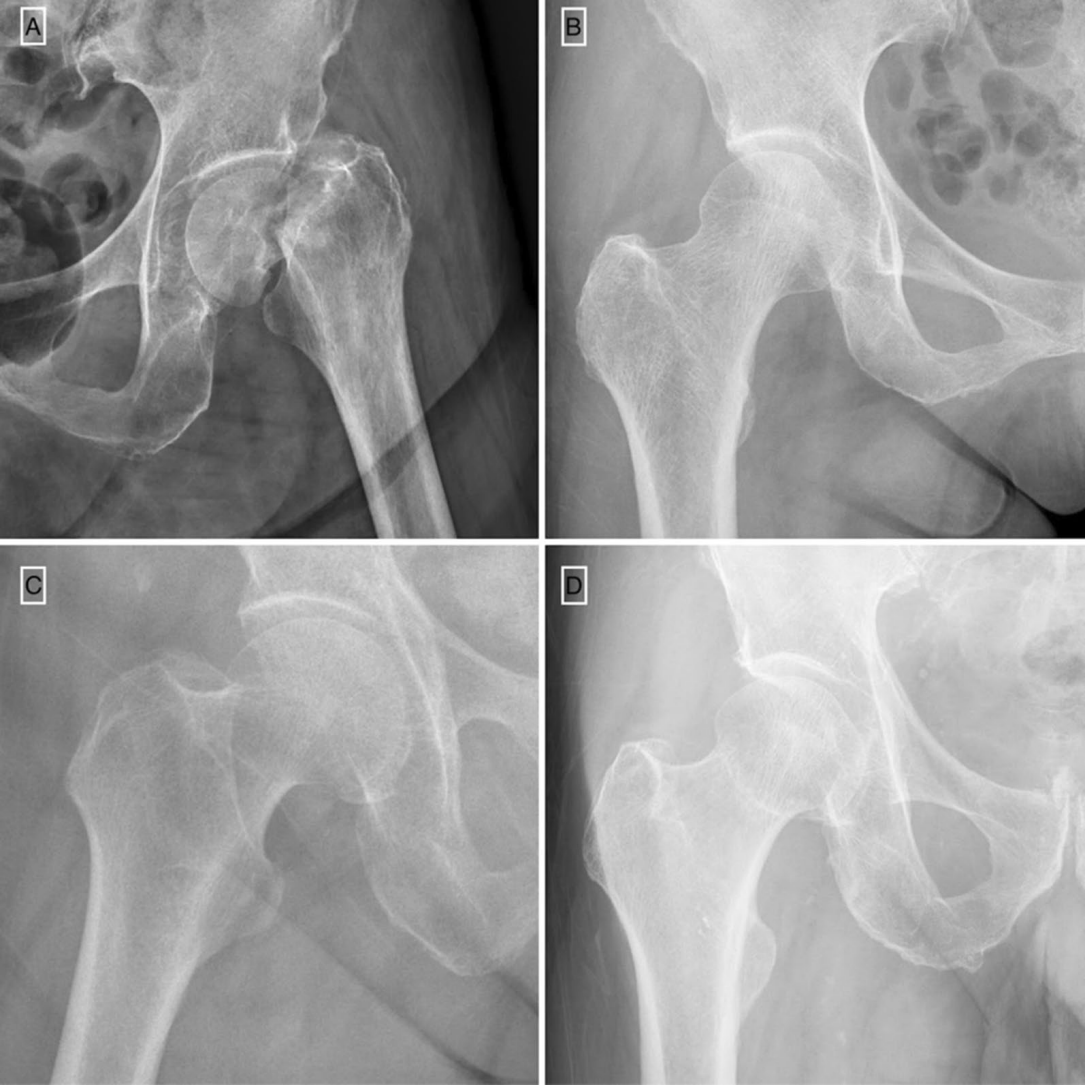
Sample X-ray images of different diagnosis rates. A: The significant hip fracture with a high detection rate of 98.3%; B The normal and healthy hip with 98.9% correct rejection; C The non-fracture hip but the sclerotic line causes a high false positive rate, 67.4%; and D An occult hip fracture without displacement. The correction detection of fracture is only 13.6%
